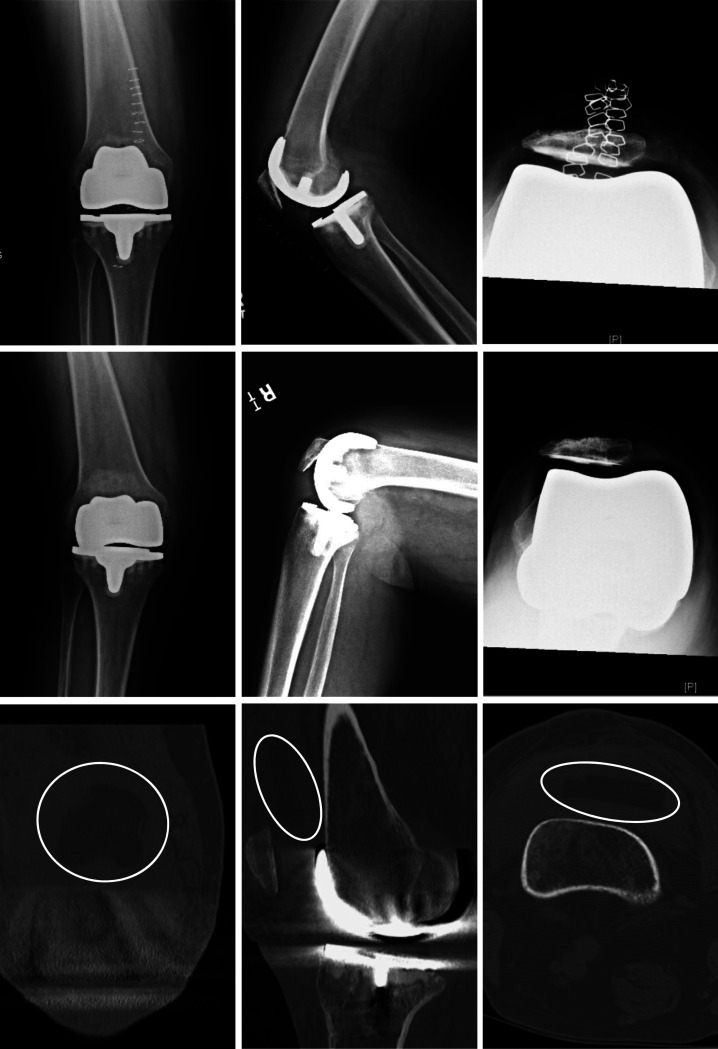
Top three images are immediate postoperative AP, lateral, and sunrise views of the right knee of a 62-year-old woman status-post total knee arthroplasty with PSD-1 implant; middle three images are 6-month postoperative AP, lateral, and sunrise views of the right knee of the same patient with lateral polyethylene dislocation; Bottom three images are coronal, sagittal, and axial CT scan images of right knee showing dislocation of lateral polyethylene insert (encircled) lodged in the suprapatellar recess
